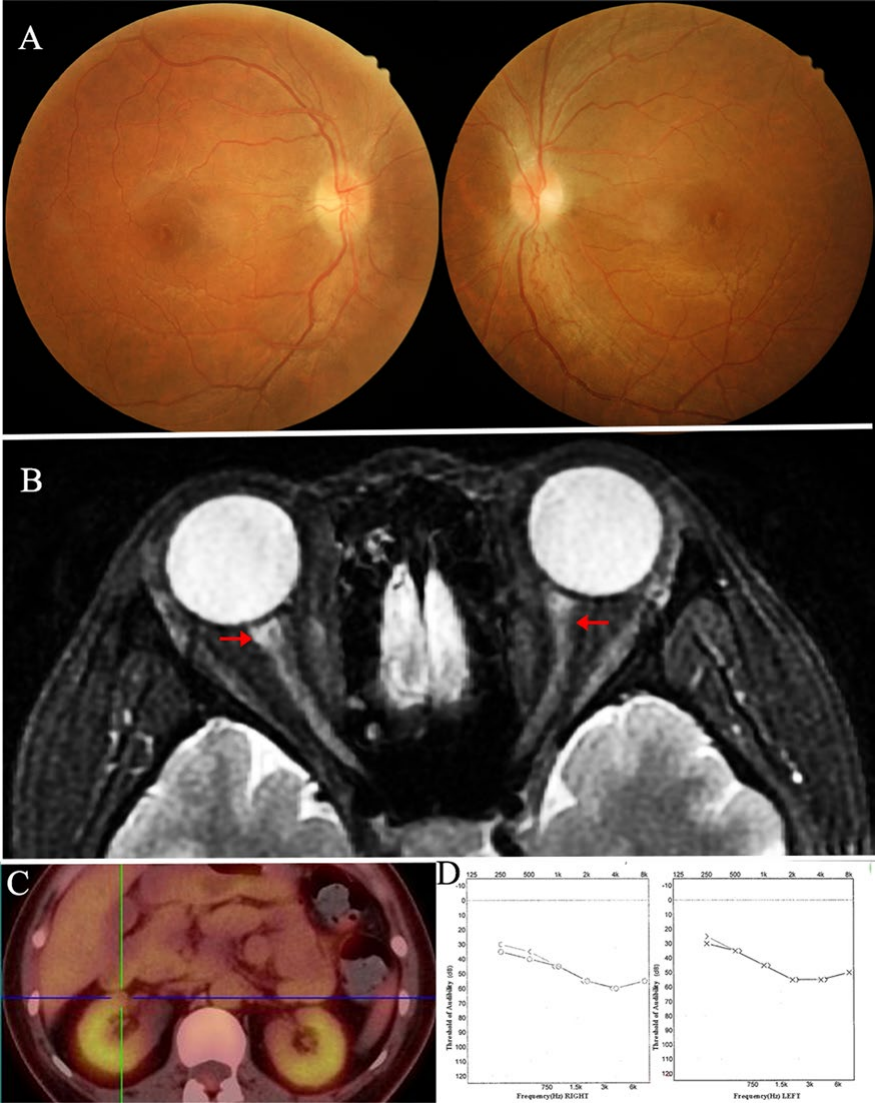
A 30-year-old woman. A, Fundus photograph: bilateral optic disc edema, copper-wiring of retinal artery, tortuosity of the central retinal artery. B, Axial T2-weighted MRI show hyperintense signal at bilateral optic nerve (red arrow). C, 18F-FDG-PET: high accumulation in the renal cortex (SUVmax:5.0). D, The threshold of audibility: bilateral moderate to severe sensorineural hearing loss
